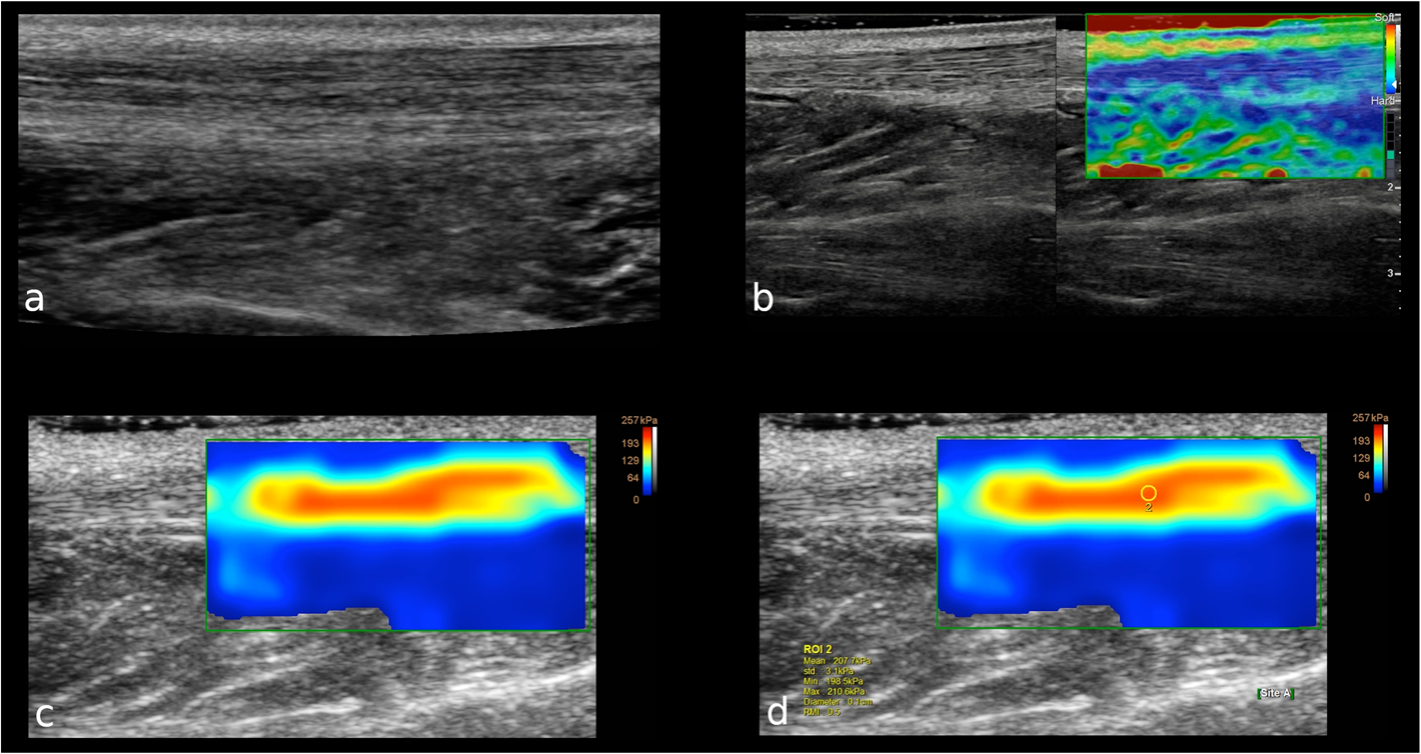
A 31-year-old male patient with healthy left Achilles tendon. a B-mode ultrasound image on longitudinal axis shows the normal thickness and echotexture of the proximal third of the Achilles tendon. b Longitudinal real-time strain sonoelastography shows the normal appearance of the proximal third of the Achilles tendon as blue, which represents stiff tissue. Subcutaneous fat tissue over the tendon appears yellow to green indicating soft tissue. c Shear wave elastography shows that the normal tendon is hard (red) and homogeneous, with the softer tissue over and below the tendon that is easy to distinguish. (d) The box is the region of interest to calculate the tendon elasticity
